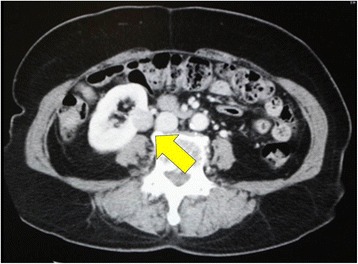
Renal cancer was suspected based on the CT findings (*arrow*)
